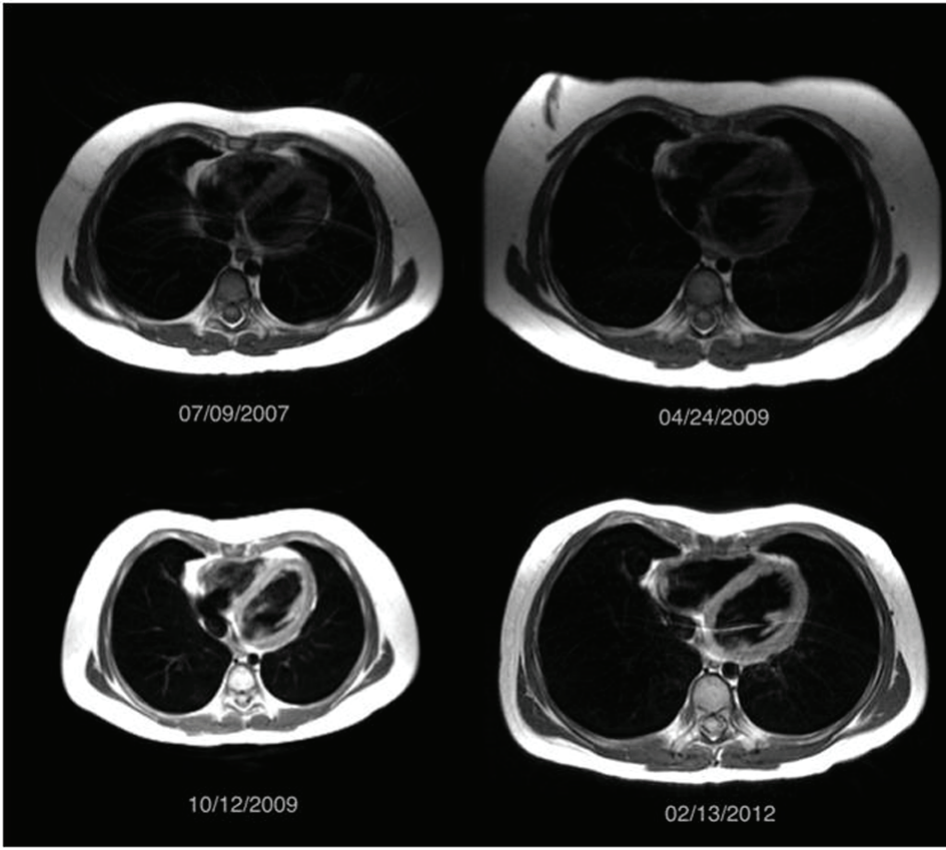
Multiple surveillance MRI scans obtained between July 2007 and February 2012 demonstrated clear lungs without evidence of an abnormal airspace filling process. The patient was asymptomatic during this time period.
